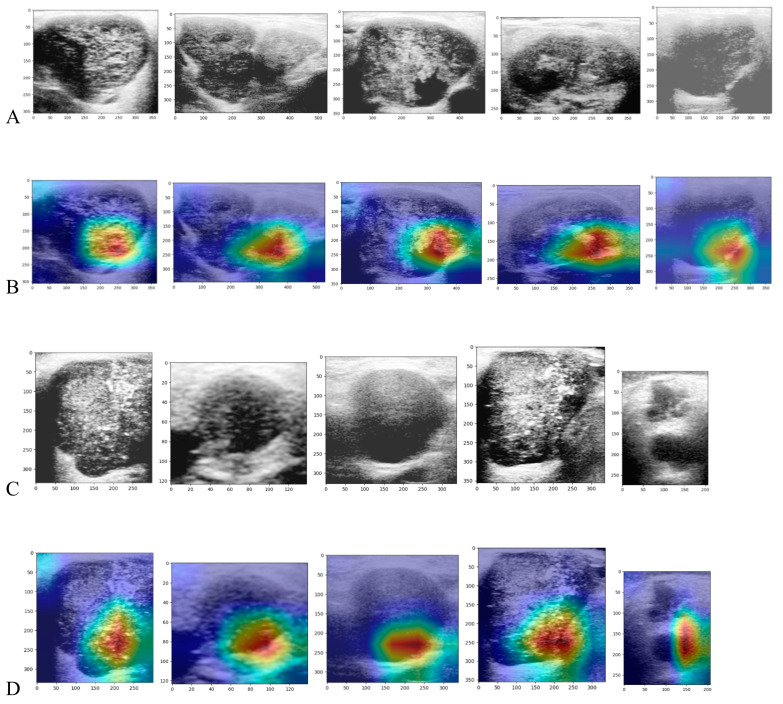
The example of ultrasound images from the testing set and its corresponding Grad-CAM image. Note: The first line displays US images of benign SGTs (A), while the second line shows their Grad-CAM images (B). The third line depicts US images of malignant SGTs (C), and the fourth line presents their Grad-CAM images (D). Abbreviation: Grad-CAM, gradient-weighted class activation mapping; US, ultrasound; SGT, salivary gland tumor.
